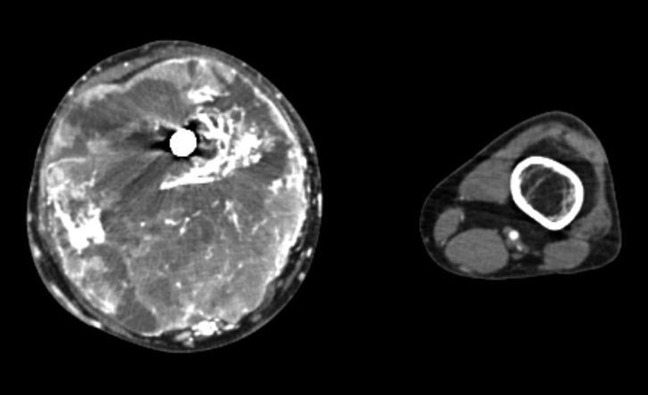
Photograph showing CT contrast-enhanced transaxial imaging demonstrating a large soft-tissue lesion with necrosis and osseous destruction of the distal femur.
